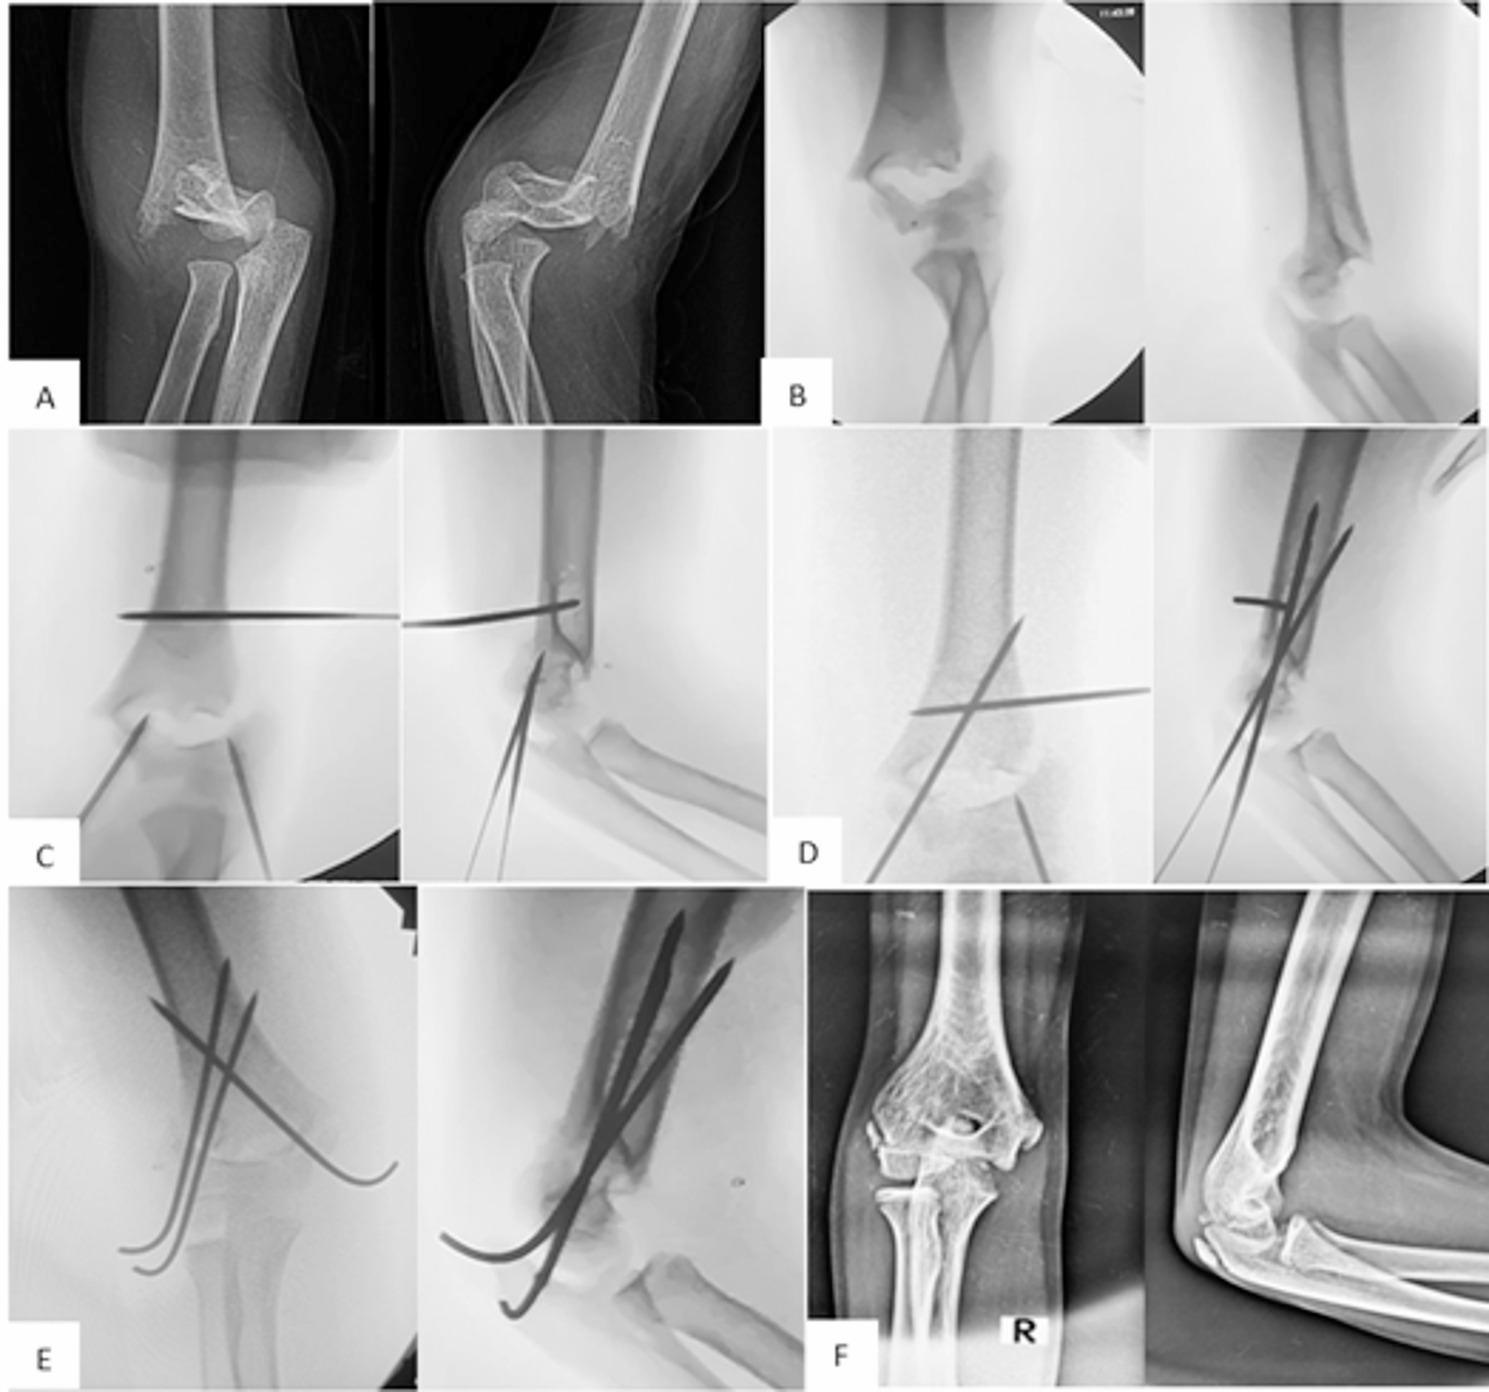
A A 13-year-old boy with displaced right-sided ESCHF. B After 2 trials of closed reduction with the traditional method. C–E Intraoperative images showing reduction of the fracture via the three-wire technique. F Follow-up plain X-rays with anterior‒posterior and lateral views showing fracture union in an acceptable position
